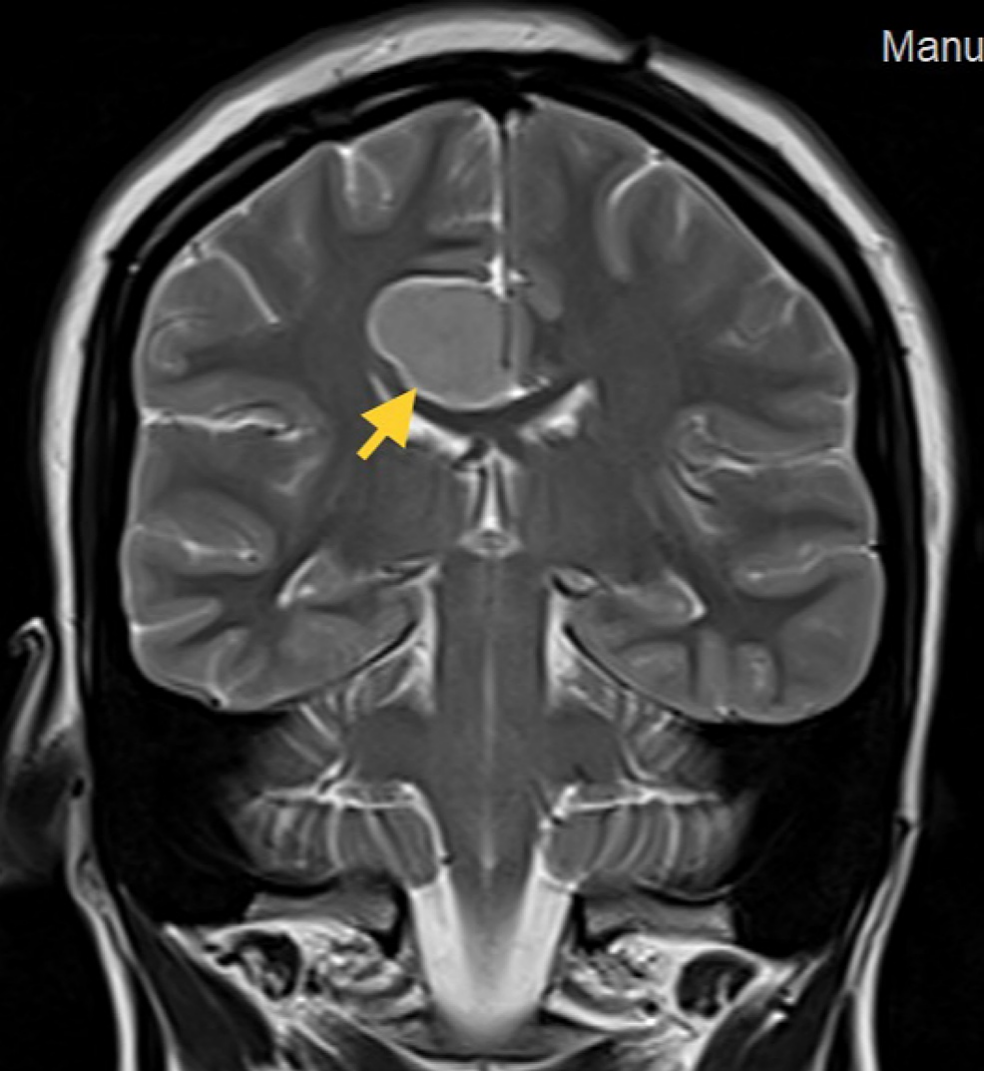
Coronal View T2 MRI with contrast showing the space-occupying lesion marked with the yellow arrow. MRI: magnetic resonance imaging
